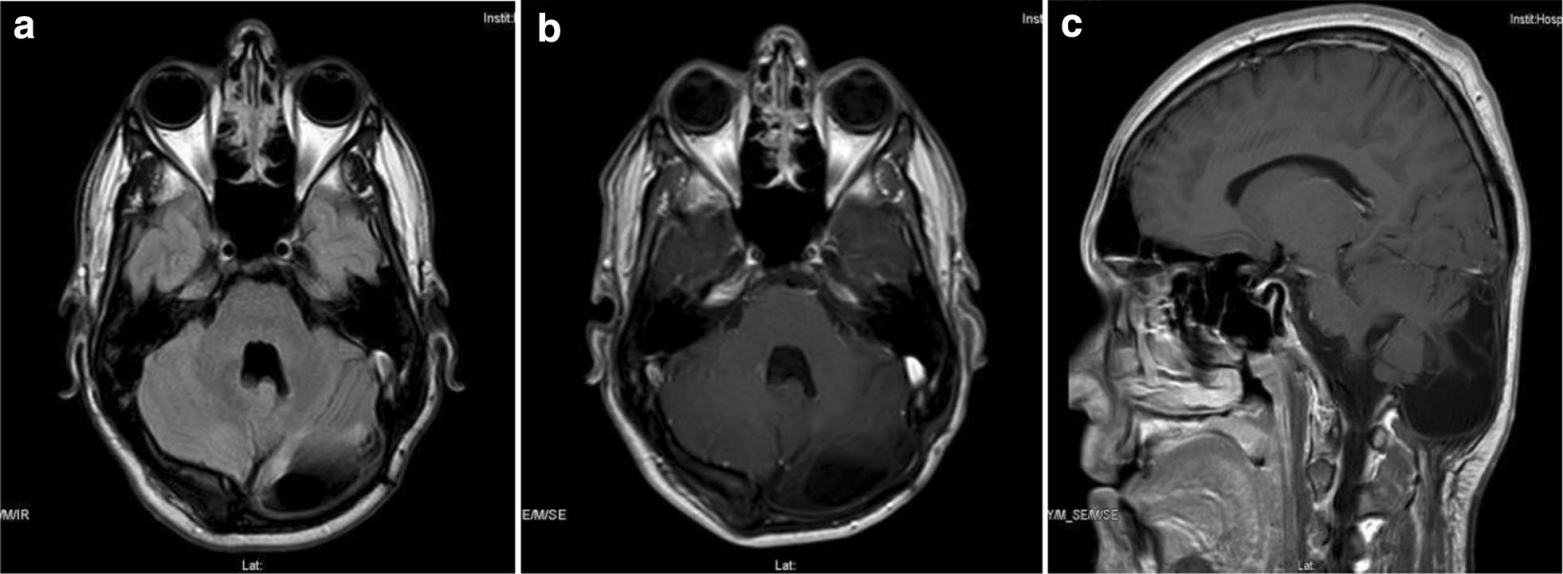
Postoperative MRI images. a FLAIR. b, c T1 gadolinium enhanced: shows complete resection of the tumor with no recurrence after 5 years follow up
